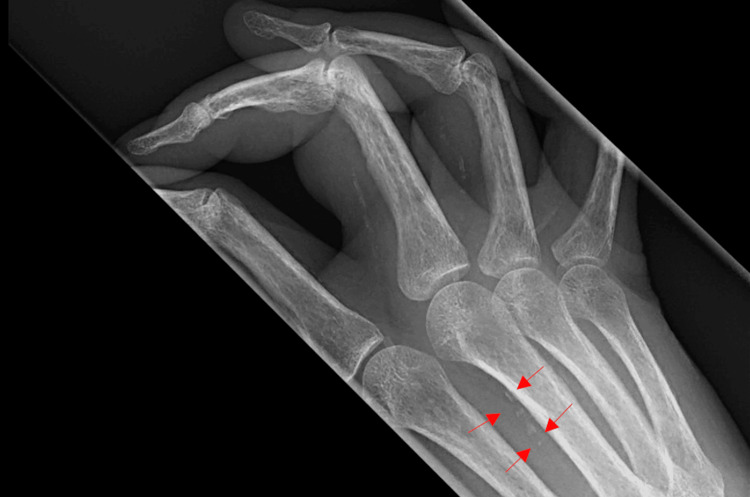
Calcification of peripheral vessels is evident on the hand X-ray lateral view (shown by arrows).
